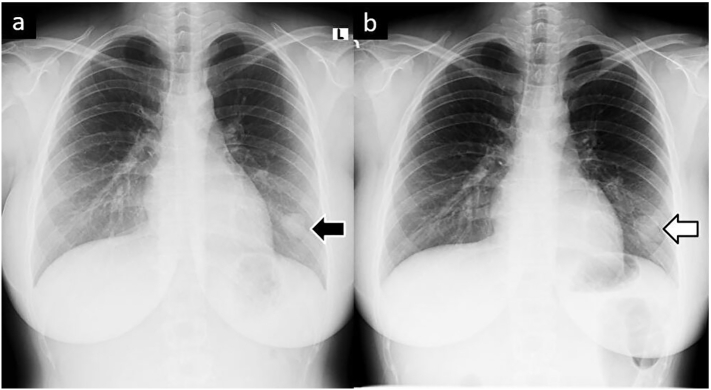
First CT after being referred to our hospital. The black arrows indicate that the original nodule had increased to 2.2 cm, and a new 1.3 cm nodule had appeared. The primary nodule had a chondrocyte component (white arrow), but the new daughter nodule did not (white triangle).
